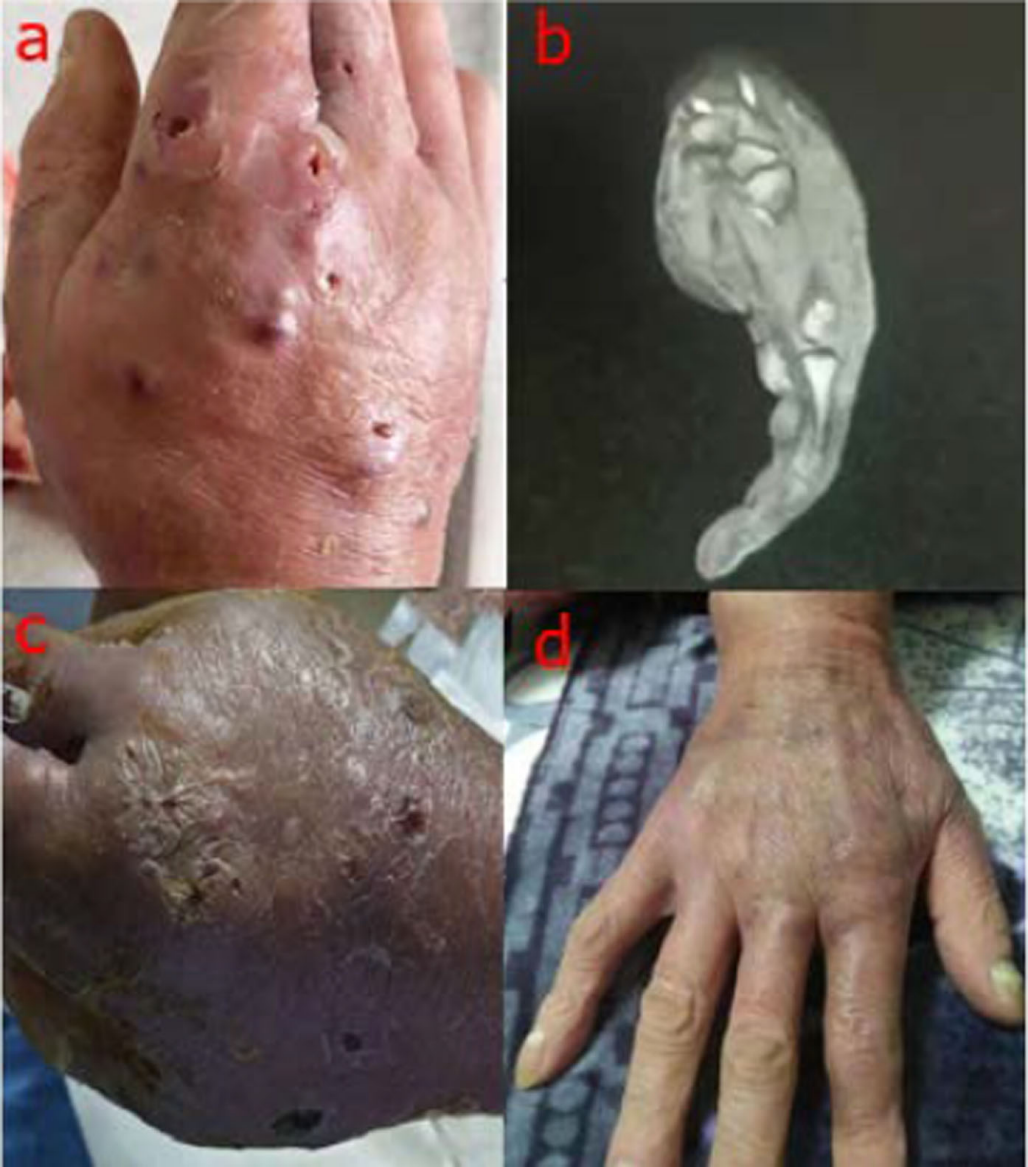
The presentation of the right hand before and after antifungal treatment in our case. a There were multiple cutaneous abscesses on the back of the red, swollen and painful right hand when the patient was admitted to our hospital. b Computed tomography scan of the hand showed the extensive tissue swelling in the wrist and palm. c After one week treatment with amphotericin B, the pus no longer discharged and the skin began to scab. d Voriconazole was administered for the patient after the isolate was identified as *E. jeanselmei*, the hand recovered very well after another two weeks
